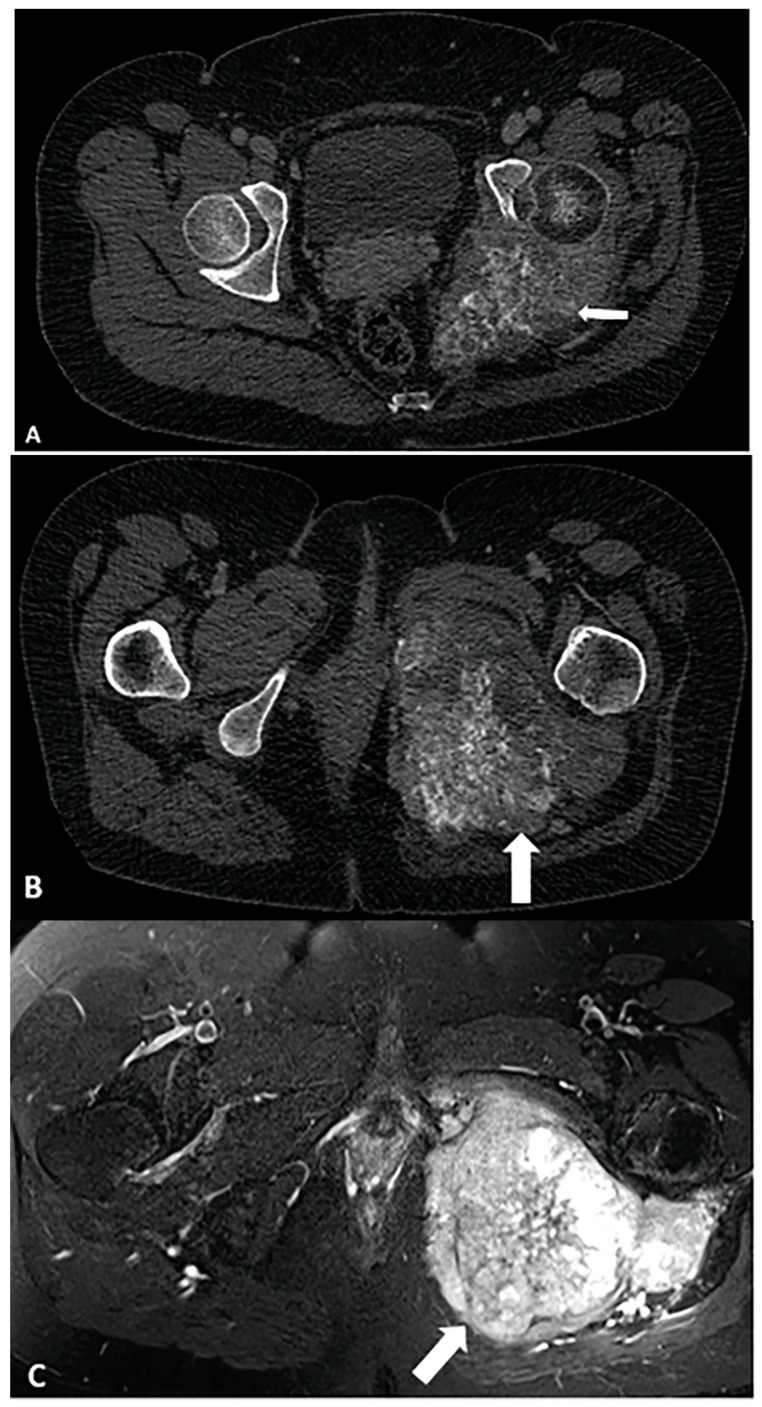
A 33-year-old female with left hip/gluteal pain. (A) CT demonstrates “rings and arcs” chondroid calcifications (arrow) and osseous destruction, findings most consistent with chondrosarcoma. (B) Contrast-enhanced CT shows enhancement in the soft tissue component of the mass (arrow). (C) Characteristic chondroid hyperintense signal intensity (arrow) is visible on a T2-weighted TSE fat-suppressed image. The image was obtained from the University of Washington Medical Imaging database with the required permissions.
